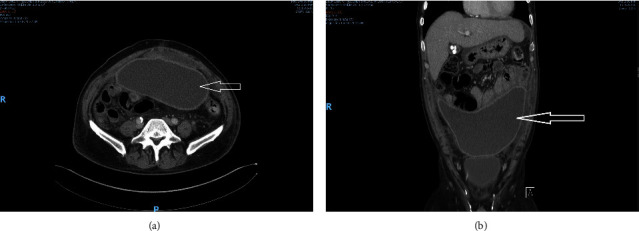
(a) Computed tomography (CT) abdomen/pelvis cross sectional view showing rim-enhancing fluid collection in the anterior peritoneal cavity measuring 22 × 13 × 7.2 cm representing intraabdominal abscess. (b) Computed tomography (CT) abdomen/pelvis coronal view showing rim-enhancing fluid collection in the anterior peritoneal cavity measuring 22 × 13 × 7.2 cm representing intraabdominal abscess.
